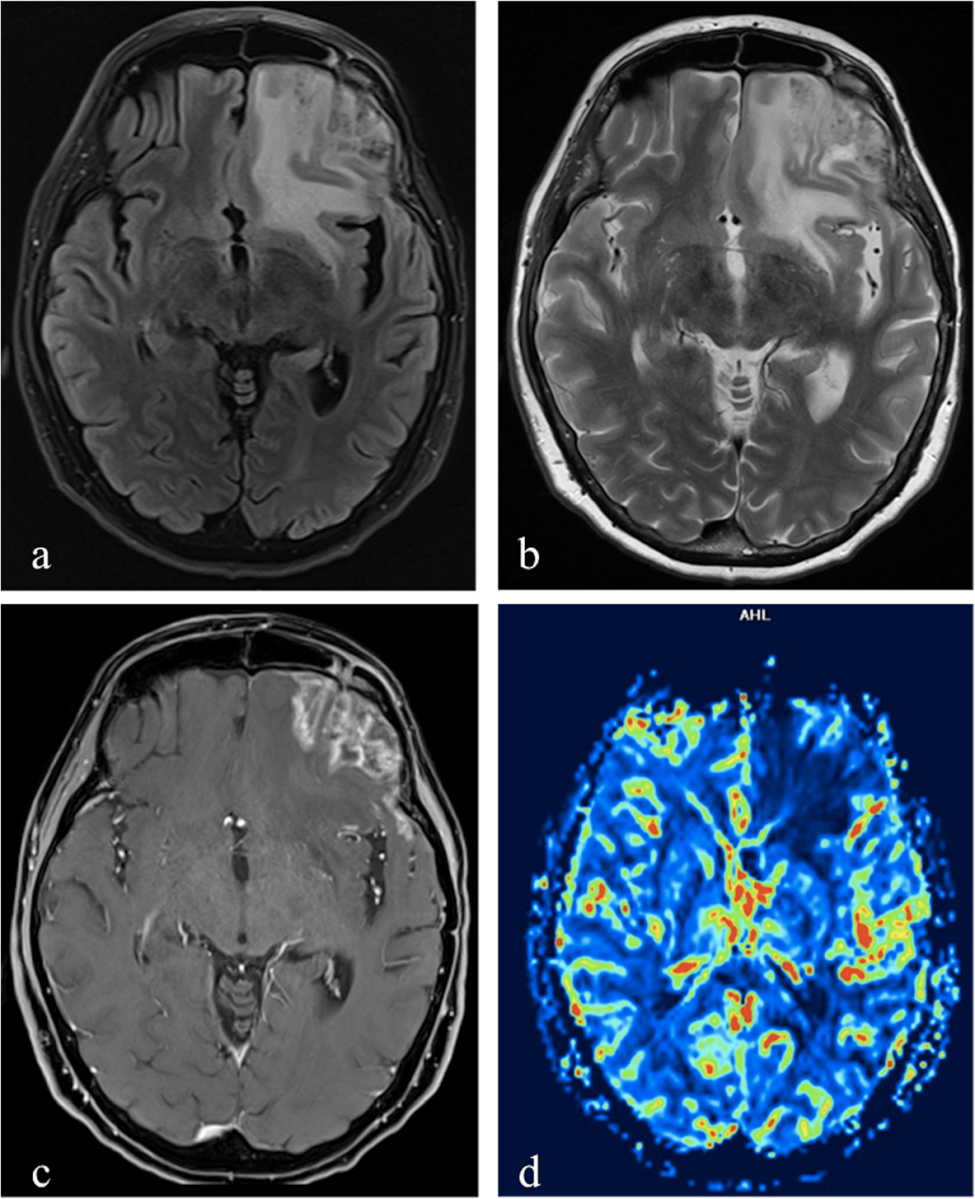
MRI performed 5 years after proton-beam therapy for skull base chondrosarcoma. Axial FLAIR (a) and T2-weighted (b) images show a disomogenous hyperintense lesion, involving both gray and white matter, in left frontal lobe surrounded by wide edematous area extending to the external capsula and causing mild mass-effect on striatum nucleus and midline structures. Axial post-contrast T1-weighted image (c) shows disomogeneous enhancement with “Swiss cheese” pattern. rCBV map (d) demonstrates no hypervascularization within the enhanced area
